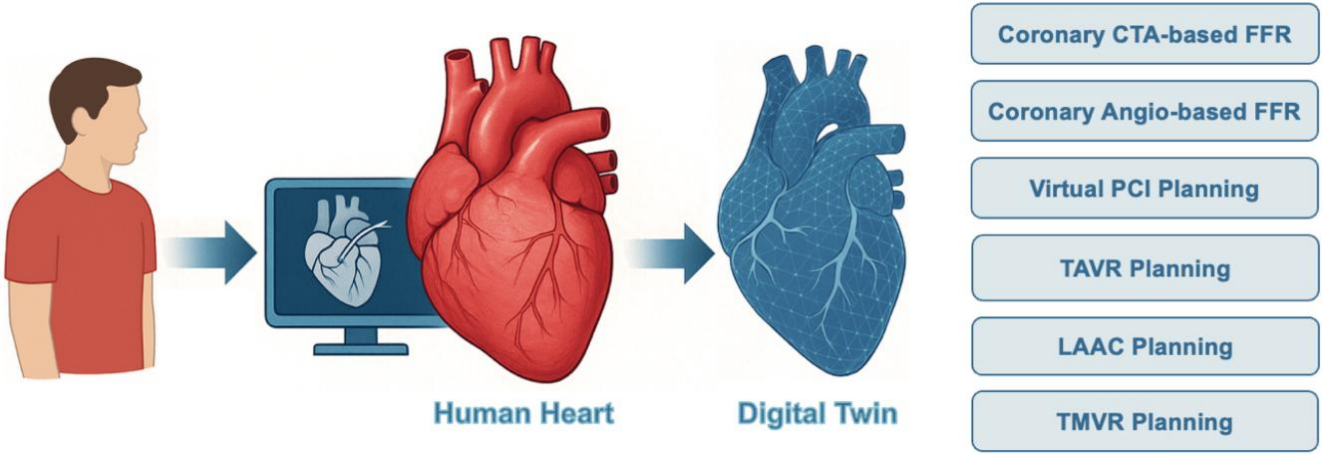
Transformation of a real heart into a patient-specific digital twin for planning of transcatheter coronary and structural heart interventions. CTA, computed tomography angiography; FFR, fractional flow reserve; LAAC, left atrial appendage closure; PCI, percutaneous coronary intervention; TAVR, transcatheter aortic valve replacement; TMVR, transcatheter mitral valve replacement.
